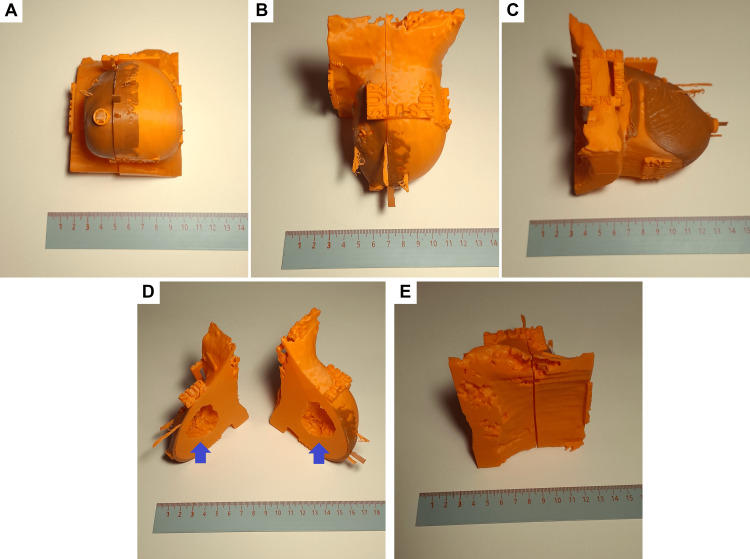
Photographs of the two-color, single-tumor breast model showing the area of skin thickening identified on MRI The area corresponding to skin thickening is printed in brown. Figure 9A shows the anterior view, Figure 9B the superior view, and Figure 9C the right lateral view. In Figure 9D, the two printed parts are shown with their inner surfaces visible. The cavity corresponding to the lesion identified as a tumor is indicated by the blue arrows. In Figure 9E, the posterior surface of the model is visible, including the imprint corresponding to the pectoralis fascia. MRI: magnetic resonance imaging
